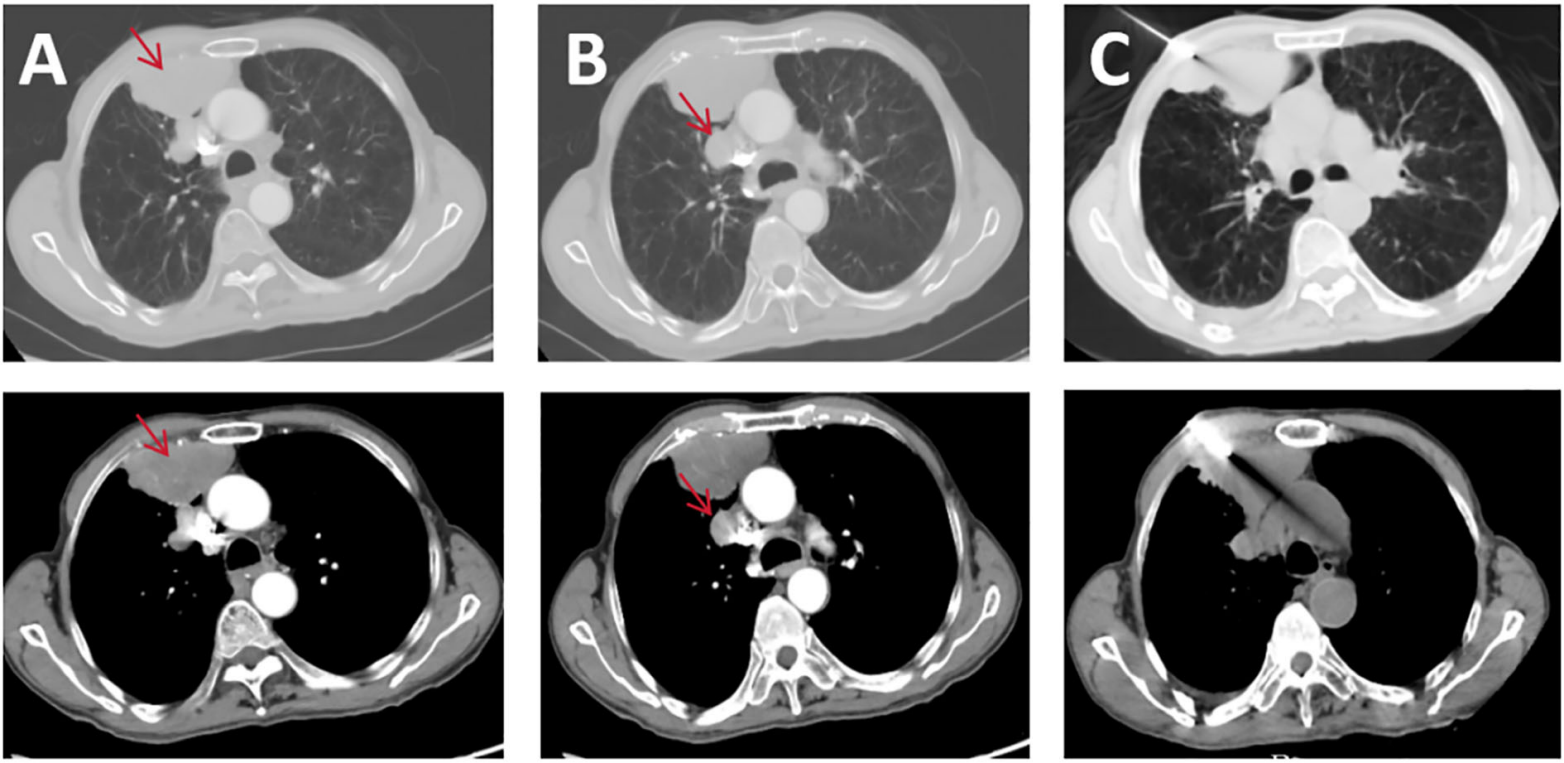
Pre-treatment CT scans: (A) pleural lesion lung window and mediastinal window; (B) mediastinal lymph node lesion lung window and mediastinal window; (C) puncture biopsy CT image.
